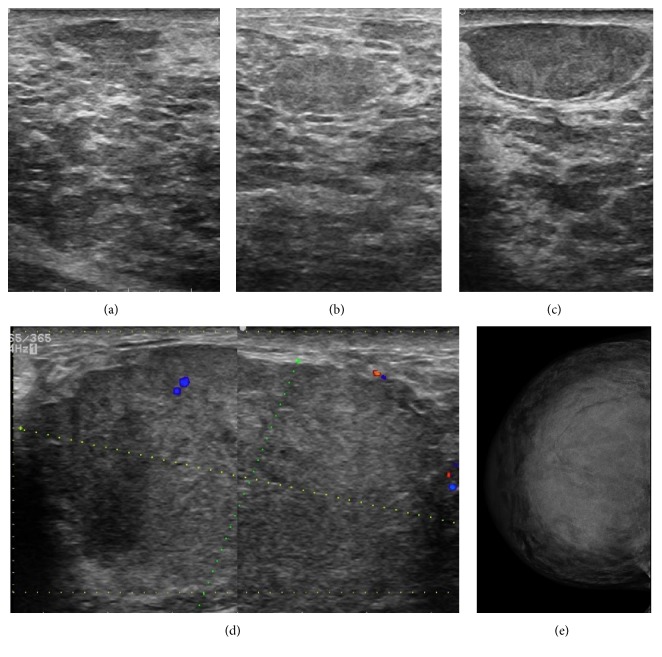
Sonographic and mammographic findings. Ultrasound of the left and right breasts showing different heterogeneous, hypoechoic, well circumscribe oval masses. Note the heterogeneous echogenicity of the breast parenchyma (a–c). Sonogram of the left breast demonstrating a hypoechoic well-circumscribed oval mass measuring 80 mm (longitudinal axis), with low flow vascularity detected by Doppler imaging (d). Craniocaudal (CC) breast mammogram of the right breast with increase in density, which decreased the sensitivity of mammography (e).
